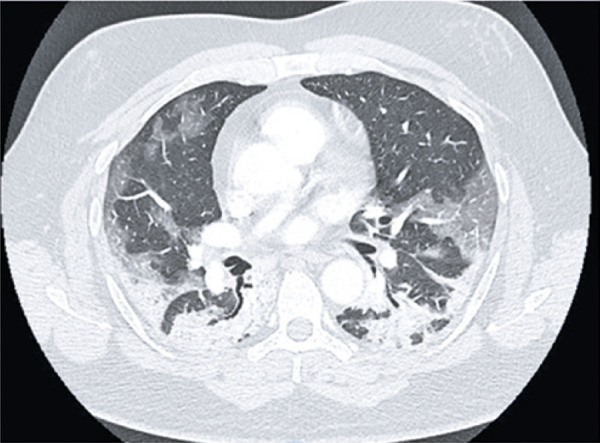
A 51-year-old patient with COVID-19, presenting fever and cough for five days. Computed tomography showing peripheral ground-glass opacities, posterior consolidations with air bronchograms
